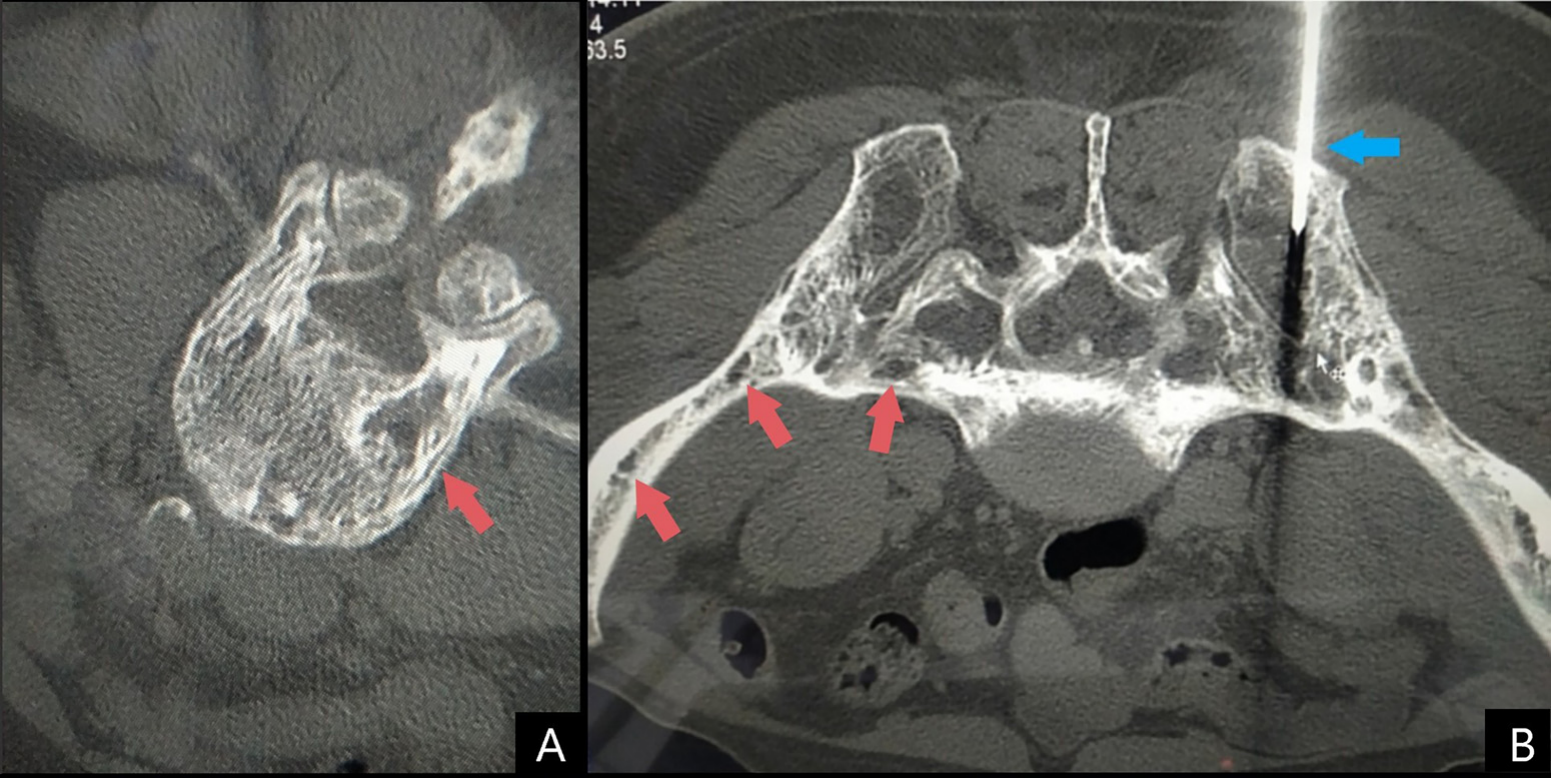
CT findings highlighting multiple lytic lesions of the spine and pelvis. (A) Lytic lesion on the L1 vertebra. (B) CT-guided bone biopsy (blue) of a lytic lesion in the pelvis. Multiple lytic lesions can be noted in the body of the pelvis and in the spine (red).
